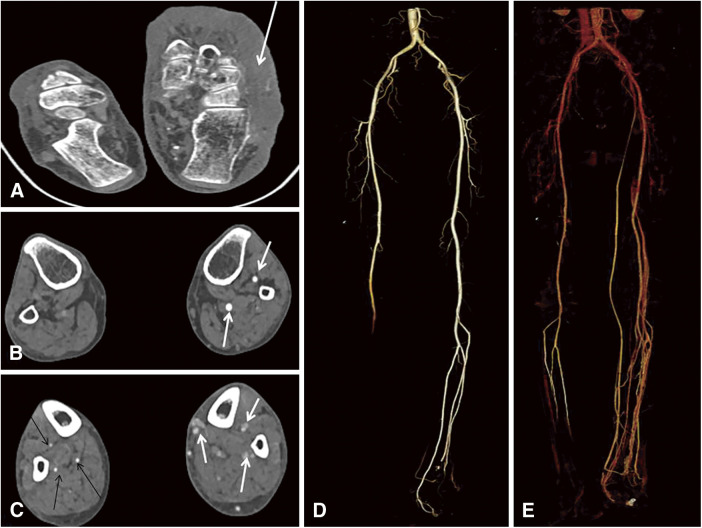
Fig. 3 (A) Axial CT image of the lower limbs at the level of the ankle shows diffuse subcutaneous edema involving the left lower leg and foot (white arrow). (B) Arterial phase CT angiography image shows luminal enhancement of the left anterior tibial and posterior tibial arteries (white arrows). There is no corresponding luminal enhancement of the right lower limb arteries. (C) The venous phase image shows good venous filling in the left lower limb (white arrows). On the right side, considerable lag is noted, with mild luminal enhancement of the right anterior tibial, posterior tibial, and peroneal arteries (black arrows). (D) 3D reconstruction of CT angiography run in the arterial phase shows a considerable lag of luminal enhancement of right lower limb arteries as compared to the left side. (E) 3D reconstruction of the venous phase run shows good venous return from the left lower limb while further distal luminal enhancement in the right lower limb arteries is noted and still lagging the left lower limb. CT: computed tomography
